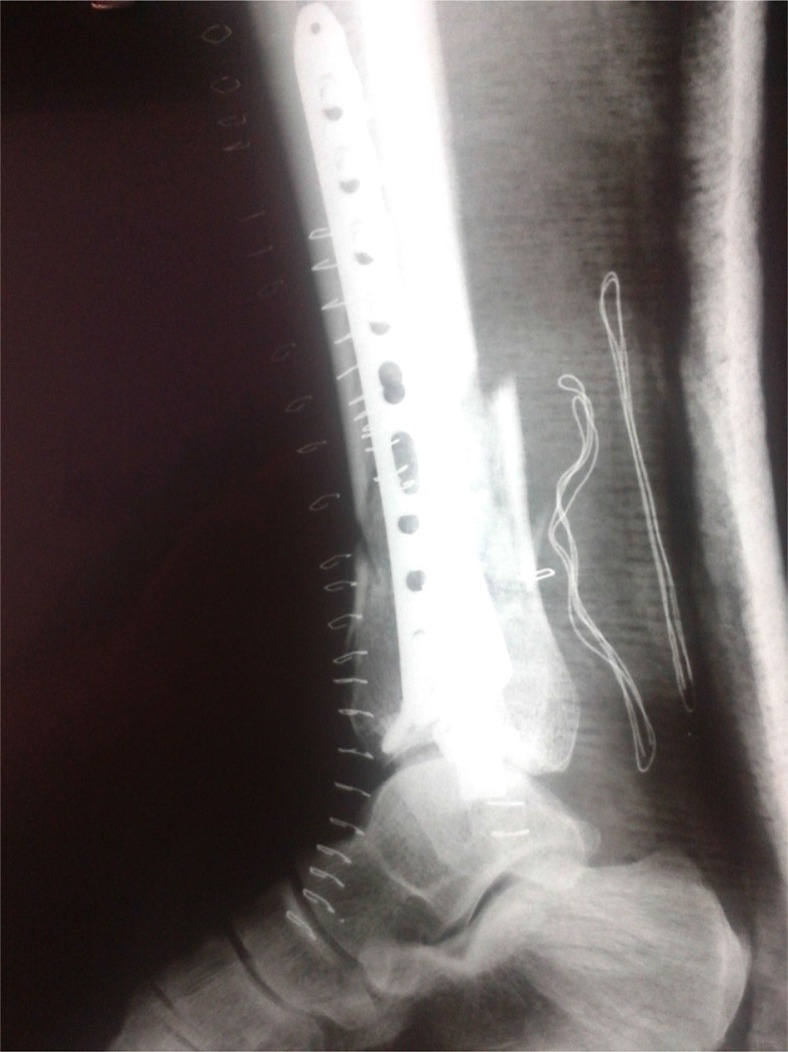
Postoperative lateral lower extremity radiograph showing the internal fixation of the pilon fracture.
